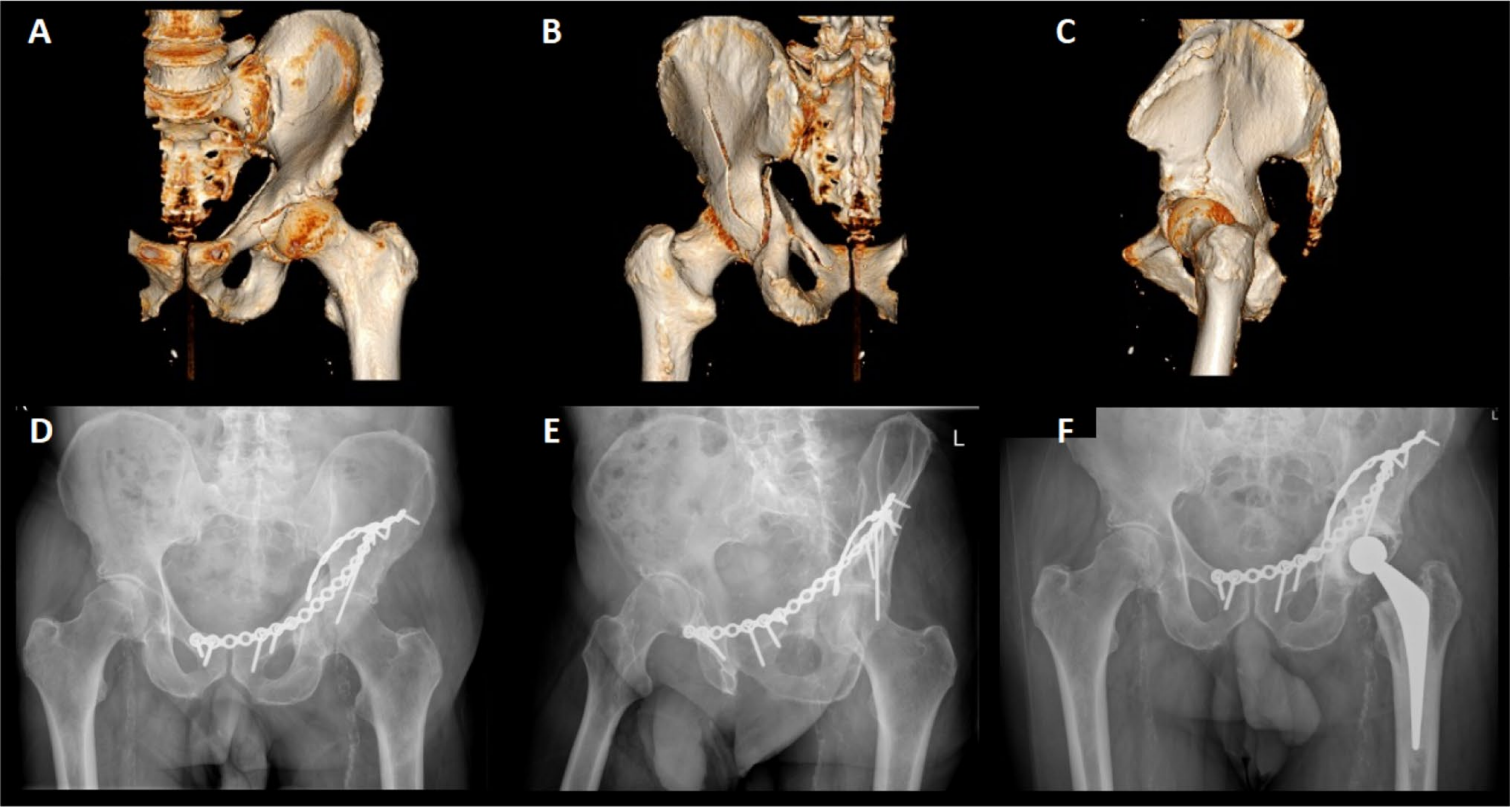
Case report: 69-year-old patient presenting with transverse posterior wall fracture following an RTA. A 3D reconstruction view (anterior view). B 3D reconstruction view (posterior view). C 3D reconstruction view (lateral view). D Plain radiograph (antero-posterior) demonstrating OA of the hip joint, with associated loss of joint space and subchondral sclerosis. E Plain radiograph (Judet obturator oblique view) demonstrating AVN of the femoral head, with loss of joint space and subchondral sclerosis, at one-year follow-up post ORIF. F Plain radiograph (antero-posterior) at five-year follow-up post THA
